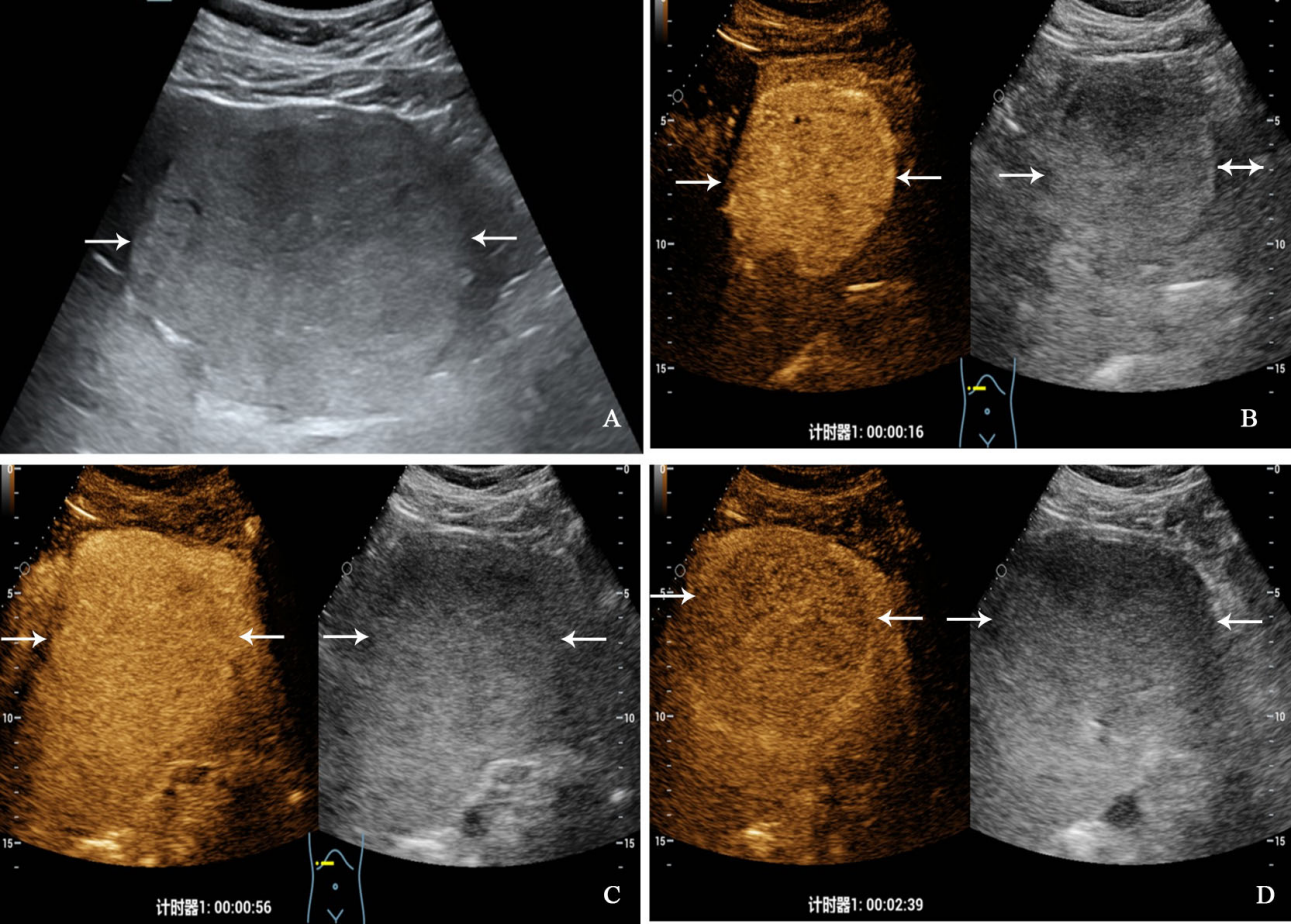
A 32-year-old man with primary neuroendocrine tumor. The patient had no underlying liver disease. Conventional ultrasound showed that a slightly hyperechoic tumor with largest diameter of 10.3 centimeters in left liver lobe (A). In the arterial phase of contrast-enhanced ultrasound, the tumor showed homogeneous hyperenhancement (B), and began washout at 56 seconds (C), hypoenhancement in the late phase (D).
